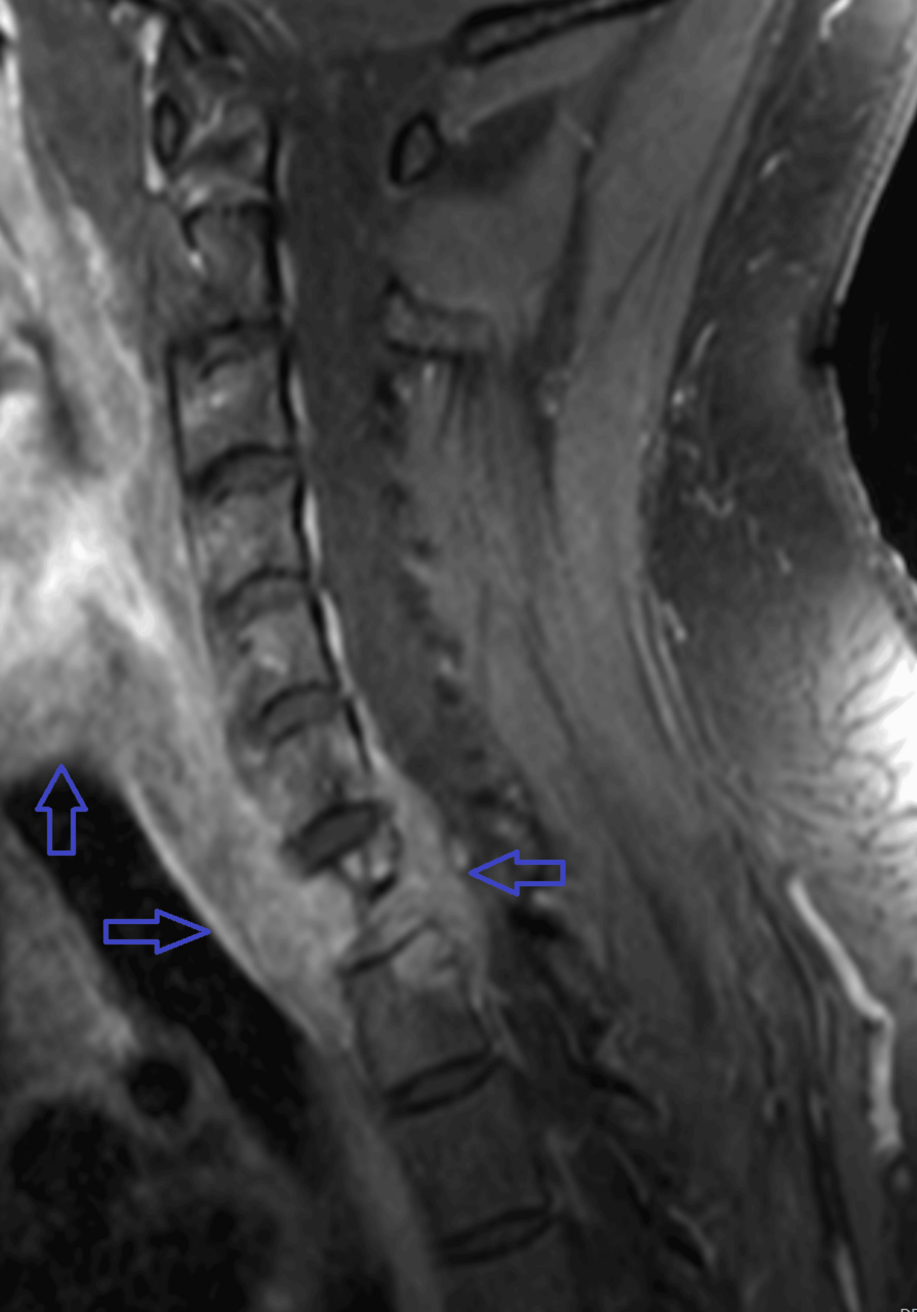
Repeat sagittal T1 post-contrast MRI demonstrating progression of the disease with near-complete destruction of the C7 vertebra due to disseminated coccidioidomycosis
